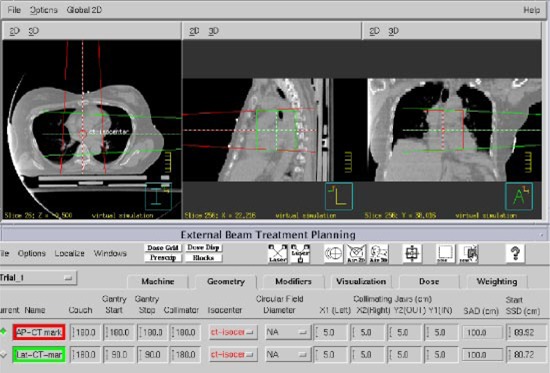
(Color) Placement of anterior and left lateral setup beams having the ‘CT Isocenter’ as their isocenter for a left breast. The anterior setup beam has an SSD=89.9 cm cm and the left lateral setup beam has an SSD=80.7 cm.
